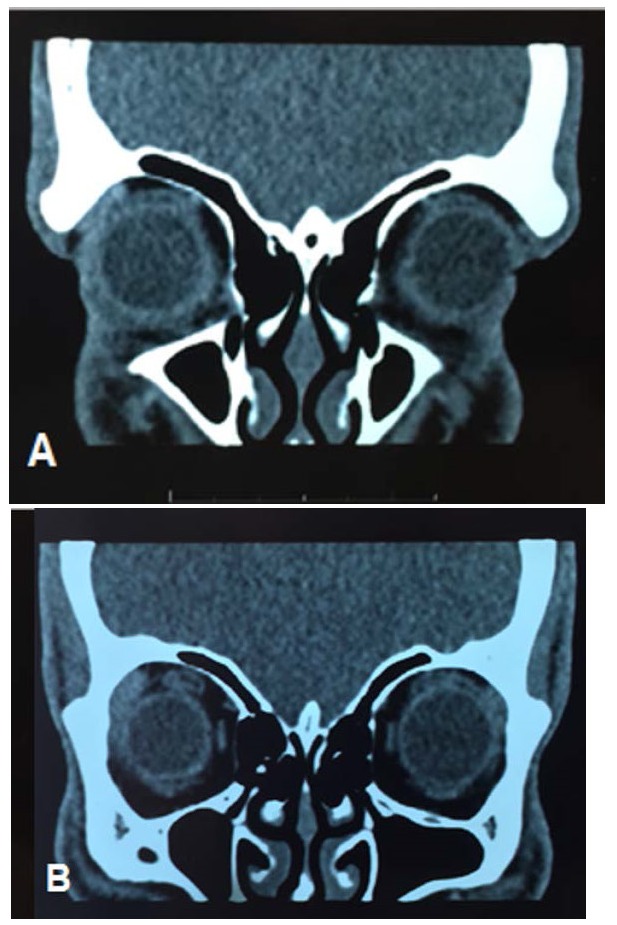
Computed tomography of orbit showing preservation of structures external to the eyeball
